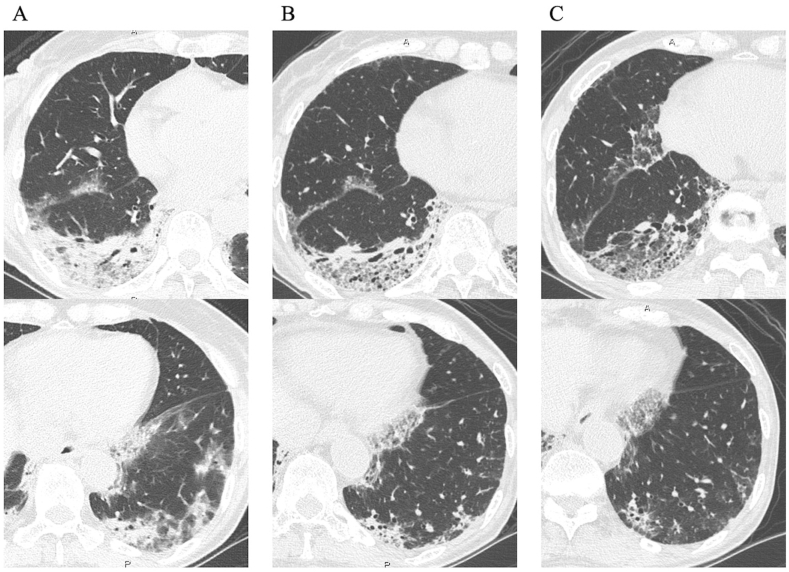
Pulmonary findings of chest high-resolution computed tomography. (A) One month after the surgical lung biopsy, bilateral subpleural consolidation and ground-glass opacity had worsened. (B) One month and (C) six months after starting the treatment, these findings had improved.
